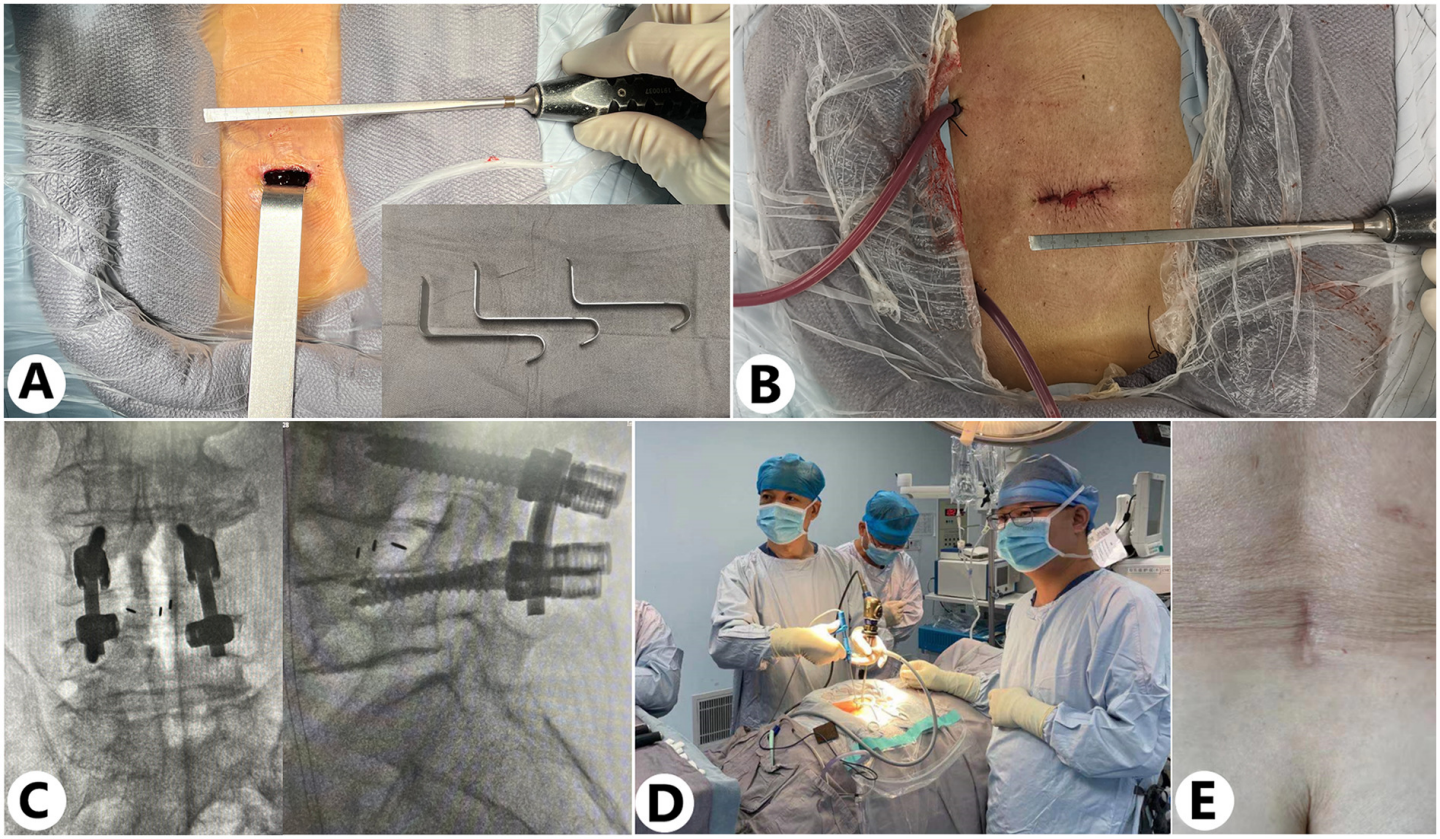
Intraoperative and postoperative imaging evaluations and surgical outcomes. (A,B) Incision size at the beginning and end of the surgery [the customized retractor is shown in the lower right corner of (A)]. (C) Intraoperative x-ray imaging was used to monitor the position of the screw-rod construct. (D) Intraoperatively, the OBE technique imaging system is used for real-time visual monitoring of manipulations in the surgical area. (E) Postoperative wound healing status.
